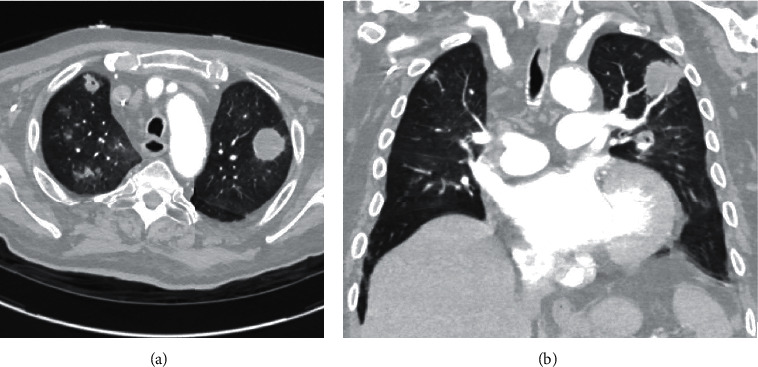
(a, b) Chest CT: invasive pulmonary aspergillosis. Chest CT findings, 2 months after initial presentation.
